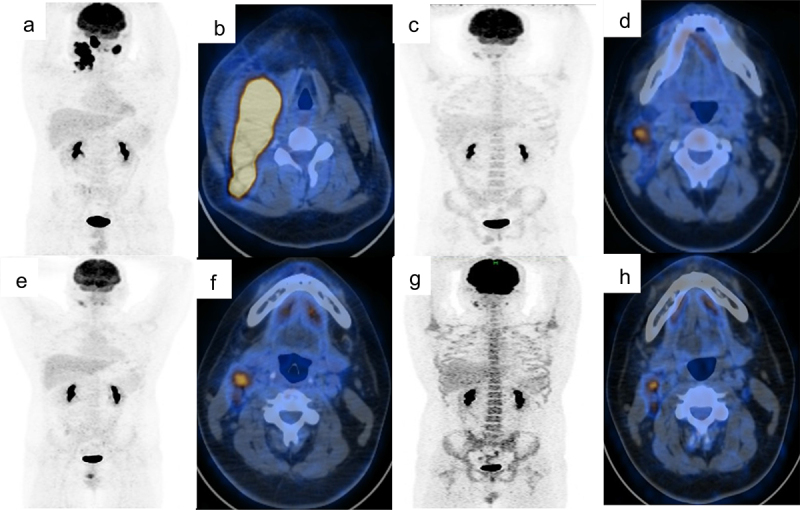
PET-CT test results before and after therapy.
